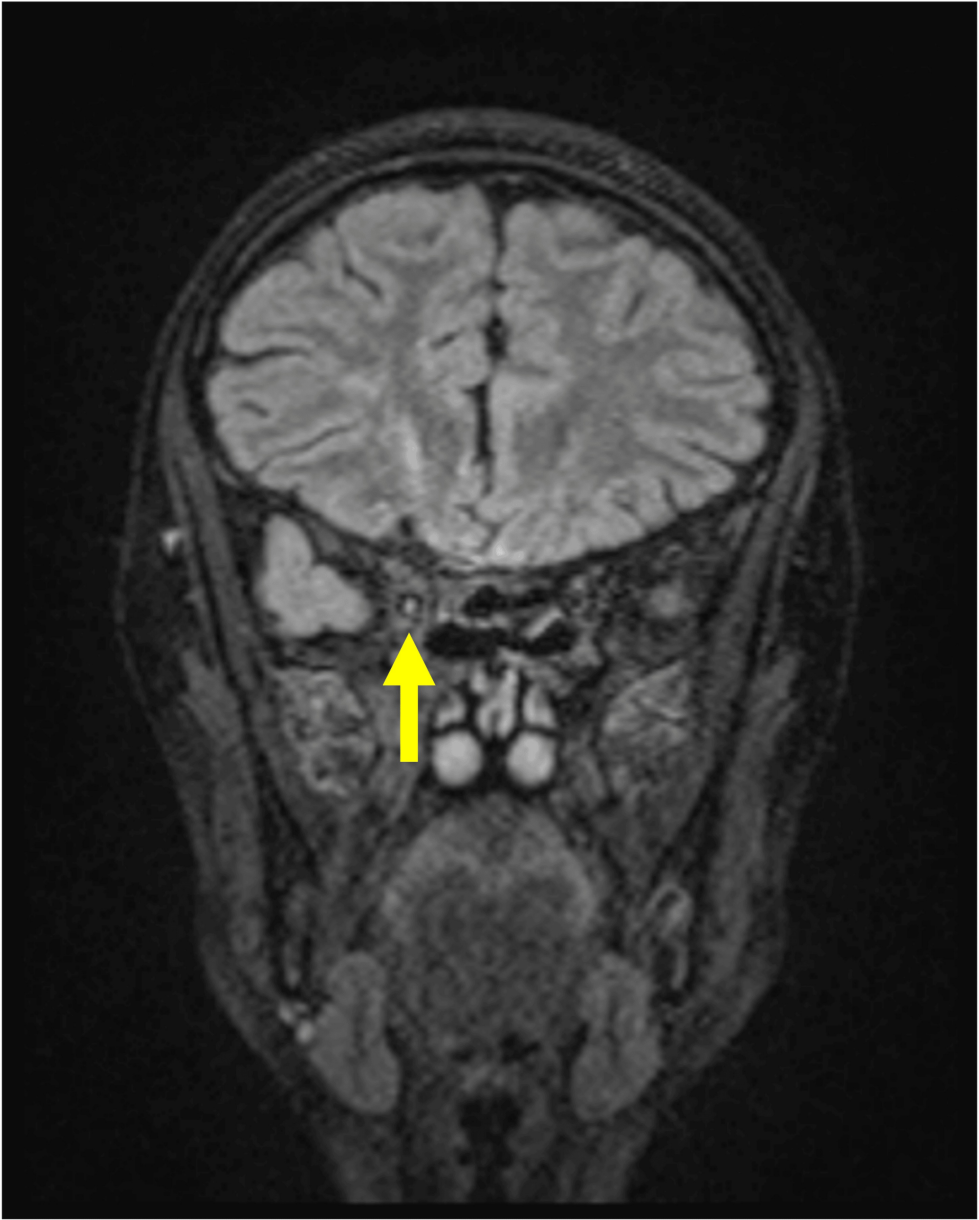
Head MRI performed approximately two months after injury A localized area of increased signal intensity suggestive of damage to the right oculomotor nerve was observed on fat-suppressed 3D-FLAIR images (yellow arrow). FLAIR, fluid-attenuated inversion recovery
